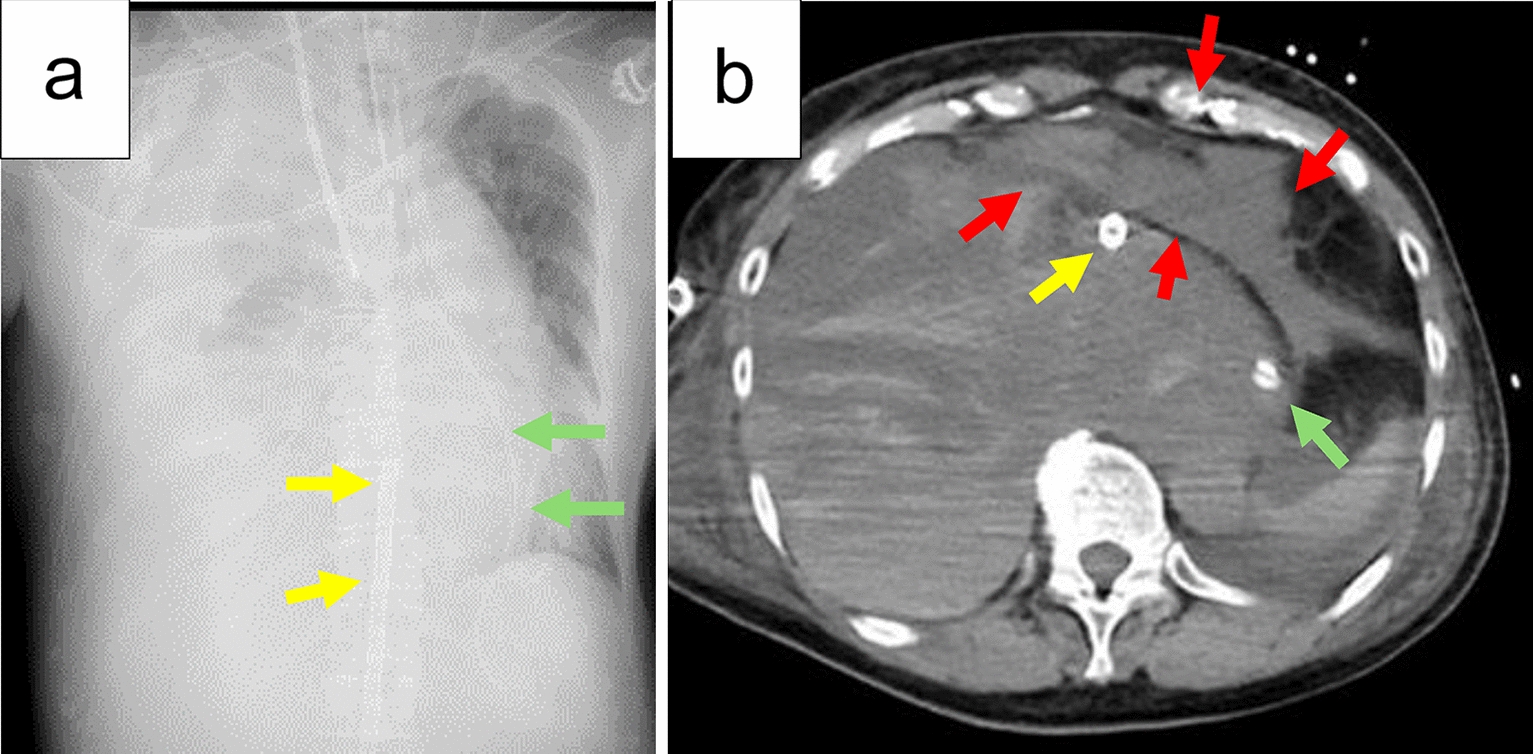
Chest X-ray and CT scan after resuscitation. a Chest X-ray showing atelectasis of the entire right lung and deviation of the mediastinum to the left. b Axial computed tomography showing massive fluid in the right pleural cavity. The heart had collapsed (red arrows). The yellow arrows show the devascularization catheter for percutaneous cardiopulmonary support in the inferior vena cava. The green arrows show the nasogastric tube in the esophagus. *CT* computed tomography
